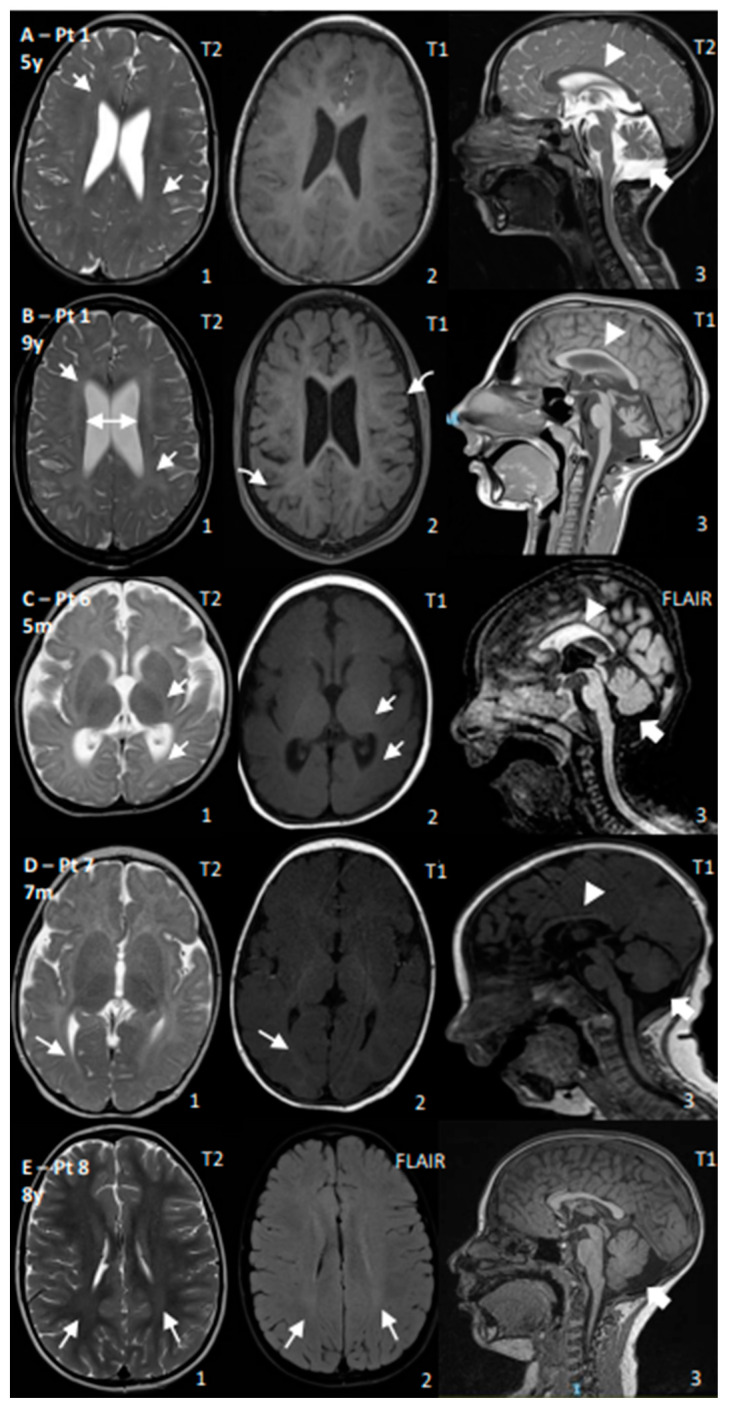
Representative brain MRI findings of cerebral and cerebellar involvement in CS subjects. (A–E): Brain MRI of patients 1 (lines A,B), 6 (line C), 7 (line D), and 8 (line E). In patient 1 with CS type I (lines A,B), thin corpus callosum (arrowheads in A3,B3), progressive cerebellar (thick arrows in A3,B3) and cerebral (curve arrows in B2) atrophy with lateral ventricle enlargement (double arrow in B1), and worsening of hypomyelination of sovratentorial white matter (thin arrows in A1,B1) are observed; hypomyelination has a patchy distribution, especially at onset, and involves both subcortical and deep white matter. In patients with CS type II (patients 6 (line C) and 7 (line D)), early reductions in brain and cerebellar volume (thick arrows in C3,D3) are present, together with a thin corpus callosum (arrowheads in C3,D3) and reductions in total amount of white matter and myelin deposit compared to age (thin arrows in C1,C2,D1,D2 indicate reduced myelination of posterior limb of the internal capsulae and optic radiation). In patient with CS type III (patient 8, line E), a mild hyperintense signal of the posterior white matter (thin arrows in E1,E2) and increase in posterior fossa with normal cerebellum (thick arrow in E3) are evident.
